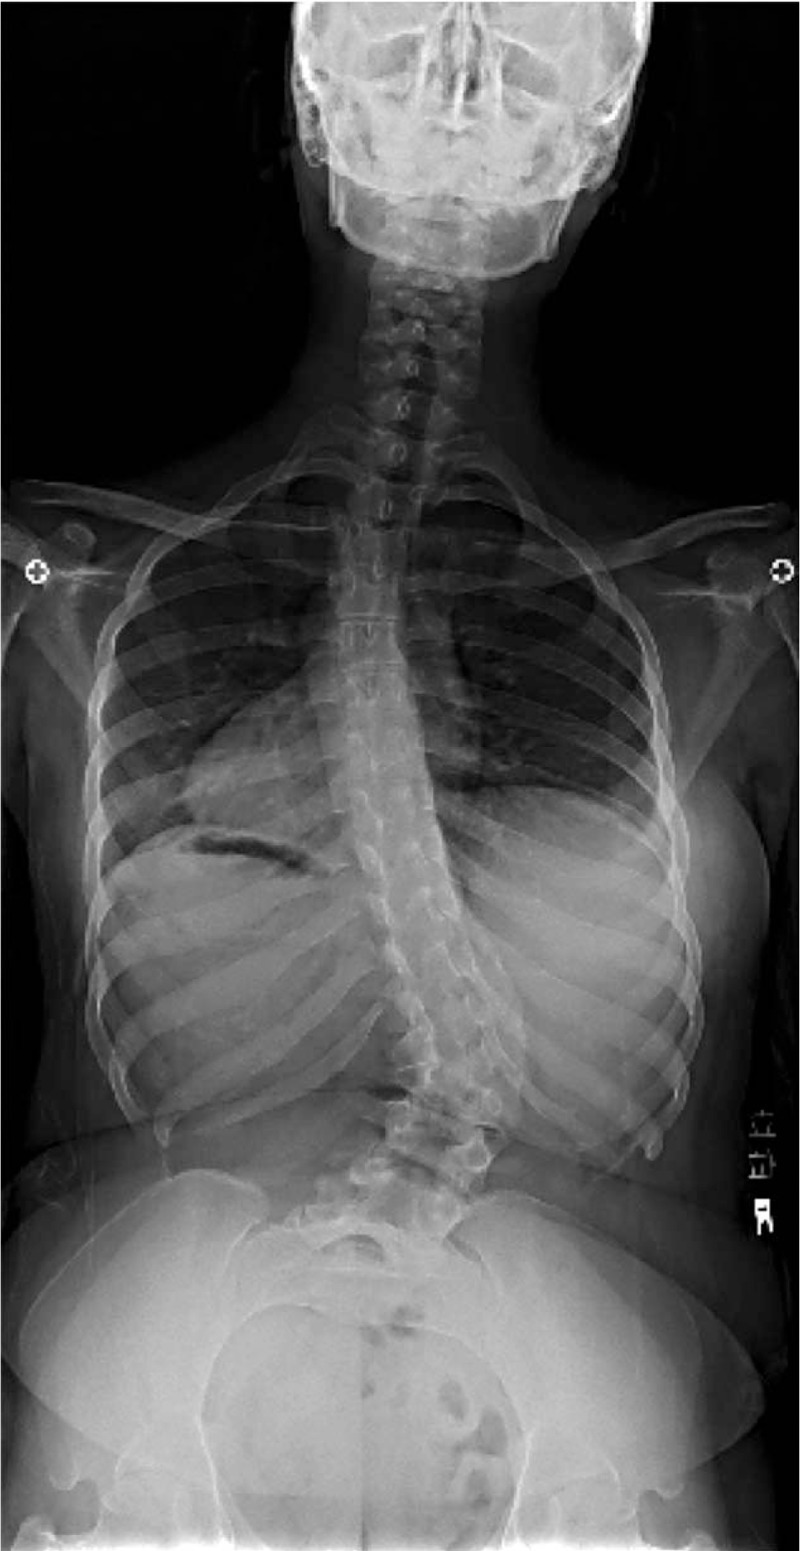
Preoperative anteroposterior radiograph showing a right lumbar curve of 41°, from T10 to L4, and a left thoracic curve of 27°, from T2 to T10.
